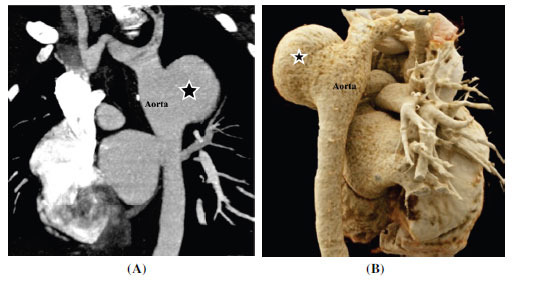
Saccular aneurysm in aortic coarctation post-surgical repair. (A) Sagittal contrast-enhanced computed tomographic angiography image showing broad neck saccular outpouching (*) of the proximal descending aorta at the site of repair of the coarctated segment. (B) Volume-rendered technique images from the back side confirm the findings.
